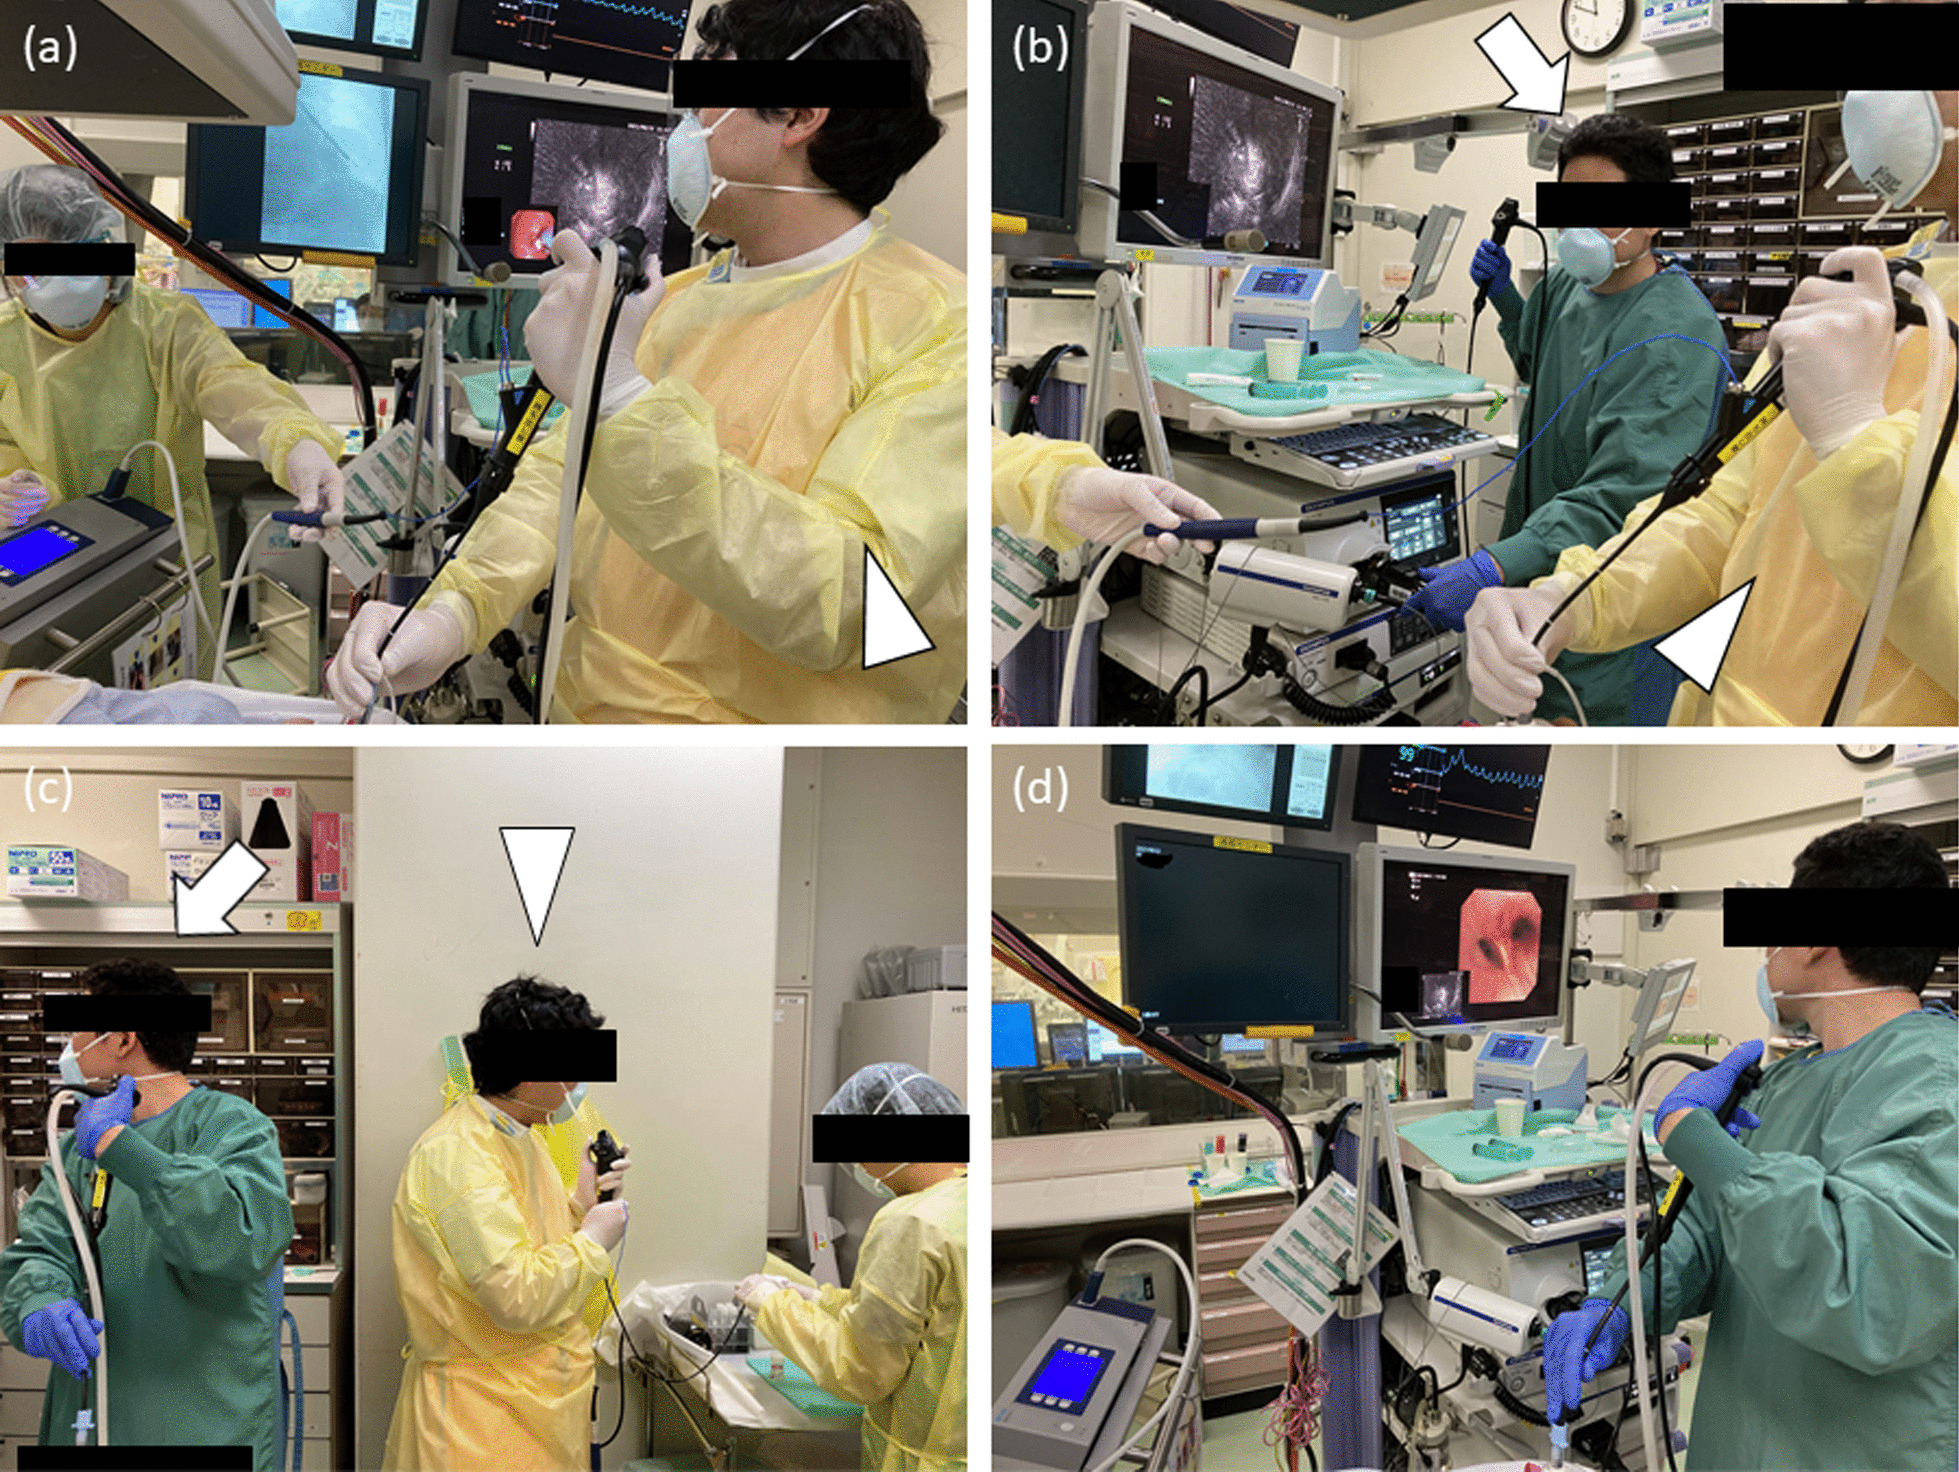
Transbronchial cryobiopsy using the two-scope technique for a peripheral pulmonary lesion. After passing the cryoprobe to the target lesion, the bronchoscope’s tip is wedged and fixed into the involved bronchus under X-ray fluoroscopy by the operator (arrowhead) (a). The bronchoscope for cryobiopsy is detached from the light source while the operator (arrowhead) keeps the cryoprobe with the bronchoscope in place, and an assistant (arrow) connects the second thick scope for hemostasis to the light source and suction tube (b). Cryobiopsy is performed under fluoroscopic guidance by the operator (arrowhead), and an assistant (arrow) immediately inserts the second thick scope through the endotracheal tube (c). An assistant controls bleeding, and the patient is simultaneously rotated to the biopsy-side down lateral decubitus position (d)
